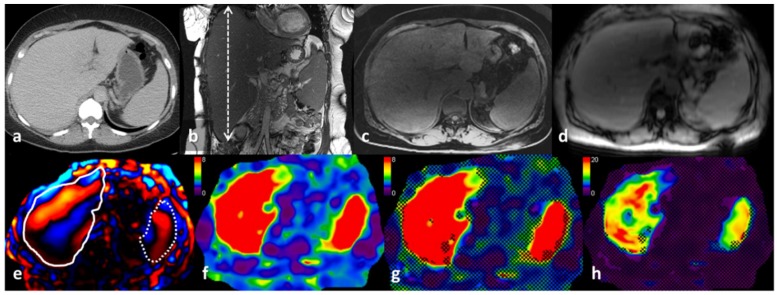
A 49-year-old female with systemic amyloidosis and hepatosplenomegaly on follow up. The axial computed tomography (CT) image (a), coronal T2-weighted image (b), and axial T1-weighted image (c) show hepatomegaly (craniocaudal span in (b), 30 cm) and splenomegaly (16 cm). Axial magnitude resonance elastography (MRE) image (d), color wave image (e), and color stiffness maps (f–h) in the upper abdomen. The liver is outlined with a continuous line, and the spleen is outlined with a dotted line on the color wave image (e). The color stiffness map (f) shows stiffness distribution on the routinely used scale of 0–8 kPa. Note that the entire liver shows an increase in stiffness. The color stiffness map (g) is the same, with a 95% confidence overlay. The regions that are not crossed out are regions with reliable measurements. Note that both the liver and spleen are almost completely outlined by the overlay. The color stiffness map (h) has a wider scale of 0–20 kPa, and one can see the heterogeneity in the distribution of stiffness. This scale is rarely used in the clinical evaluation of liver fibrosis. The mean ± SD for LSM is 18.1 ± 3.4 kPa, and for SSM, it is 16 ± 2.1 kPa.
